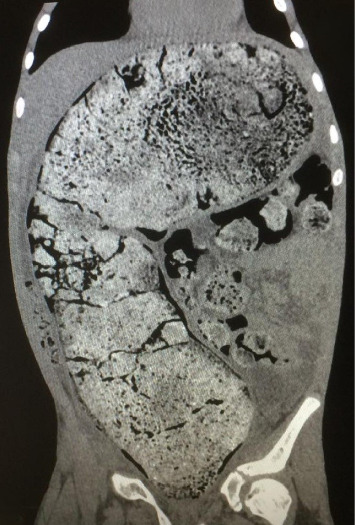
Coronal view of the abdominal CT scan, showing a distended sigmoid colon filled with large fecal masses with a maximum diameter of 11.58 cm.
